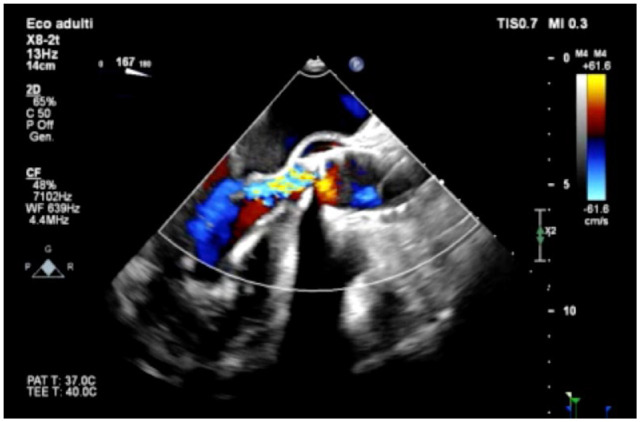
Post–transcatheter aortic valve implantation procedure transesophageal echocardiography showing significant aortic regurgitation due to the embolized bioprosthesis.
